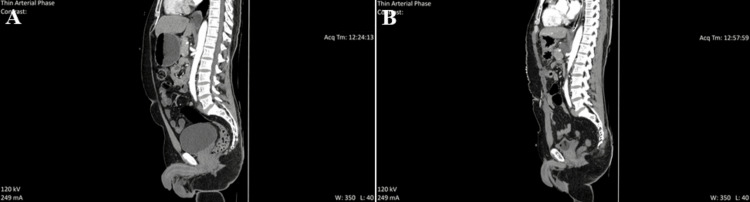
Preoperative and postoperative CT angiographic findings in median arcuate ligament syndrome. (A) Preoperative sagittal CT angiography demonstrating characteristic focal proximal celiac artery narrowing with a hooked appearance due to external compression by the median arcuate ligament; and (B) postoperative CT angiography demonstrating restoration of the celiac artery lumen following successful laparoscopic median arcuate ligament release.
